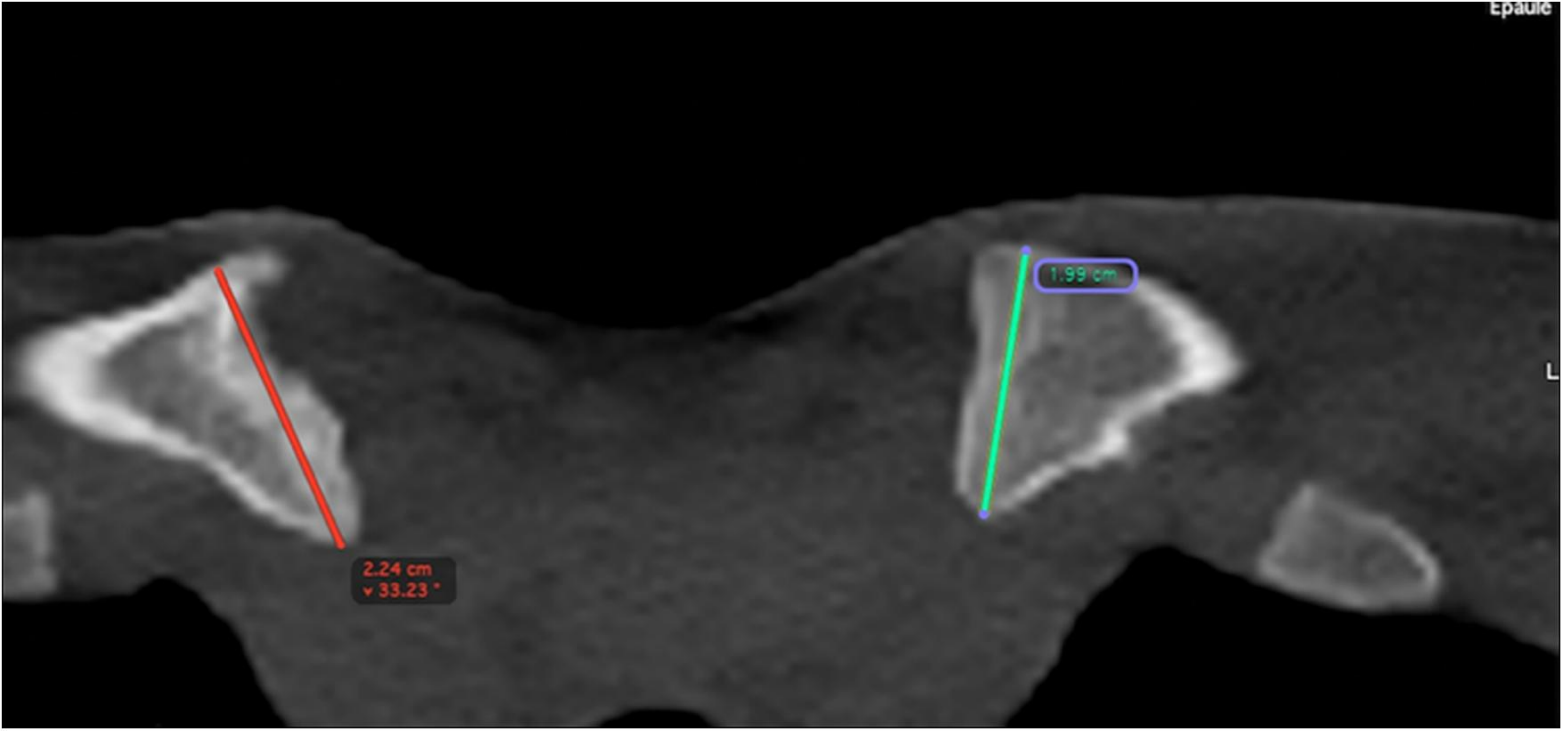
CT showing the irregularity of the metaphysis with enlarged aspect (red line).
